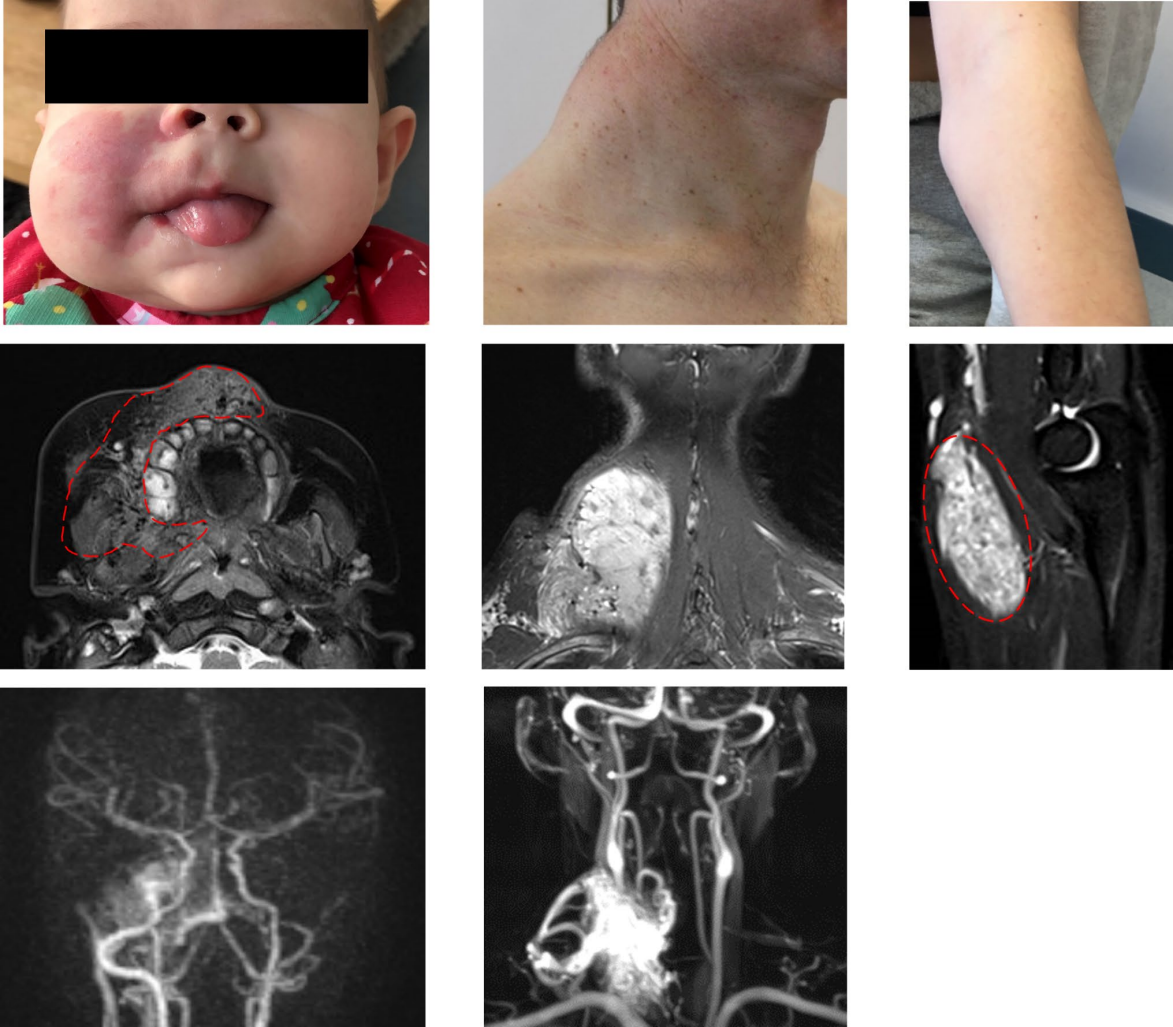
Three patients with somatic *RIT1* variants identified in AVM tissue. a Patient P1 displays a capillary malformation and swelling of the right side of the face. b MRI of P1 at the age of 4 months, the image of a transversal T2 TSE sequence, in which an AVM could be detected; the extent of the lesion is labelled with red dashed line indicating soft tissue edema and flow-voids. c The MR angiography shows increased perfusion on the right side of the face (left side within the panel). d Patient P2 shows a prominent mass of the left cervical/nuchal area. e MR imaging (coronal T2 sequence with fat saturation), which shows the hyperintense isolated intramuscular lesion, flow-voids seen within the lesion representing high AVM flow, and disfiguring overgrowth. f MR angiography, which shows the large AVM connected to the subclavian and the thyrocervical trunk with multi-chambered central nidus. g Clinical aspect of the Patient P3 with swelling on the left forearm, close to the medial side of the elbow. h T2W sagittal images demonstrating a well-defined fusiform shaped hyperintense lesion involving the flexor muscle (pronator teres) of the forearm. Flow voids (red dashed line) are seen within the lesion representing arterial blood vessels. *MRI* Magnetic Resonance Imaging
